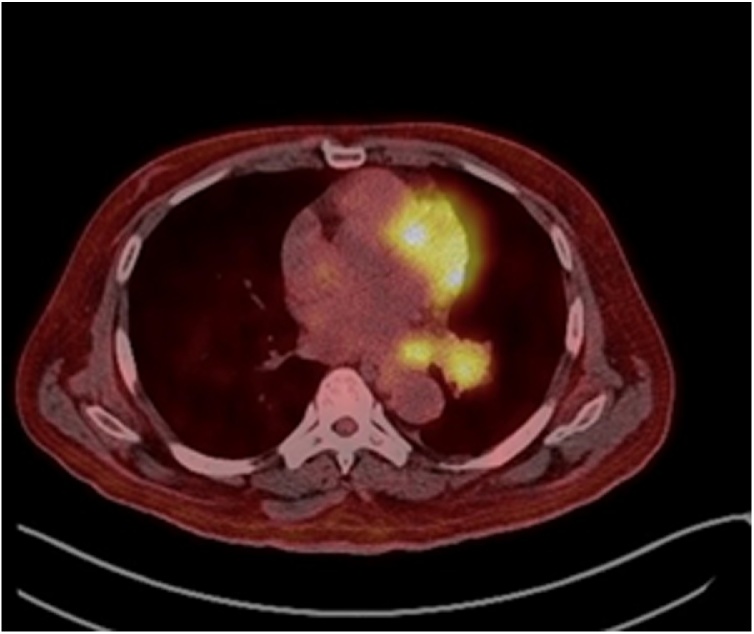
FDG PET scan axial view showing left parahilar mass in the left lower lung lobe most likely lung cancer.
